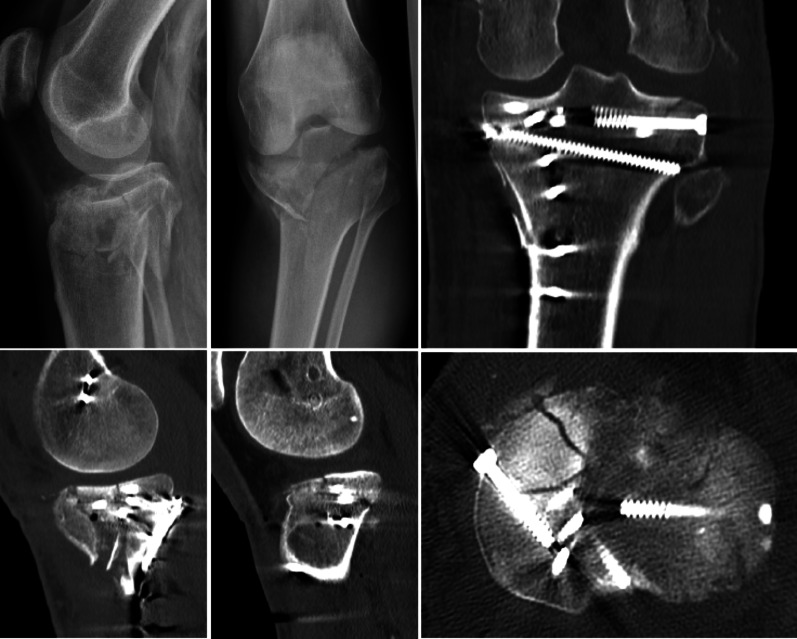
Pre- and postoperative imaging of a bicondylar tibial plateau fracture treated with osteotomy of the medial epicondylus. 42-year-old carpenter with hyperextension tibial plateau fracture with an acute compartment syndrome without neurovascular injury after a 150 kg pillar hit his upper thigh. A–B: Preoperative presentation of an AO/OTA 41-C3-3 bicondylar tibial plateau fracture (A: sagittal, B: coronar). All 10 segments are involved [12]. C–F: Post-operative CT control after anterolateral and medial plate osteosynthesis
